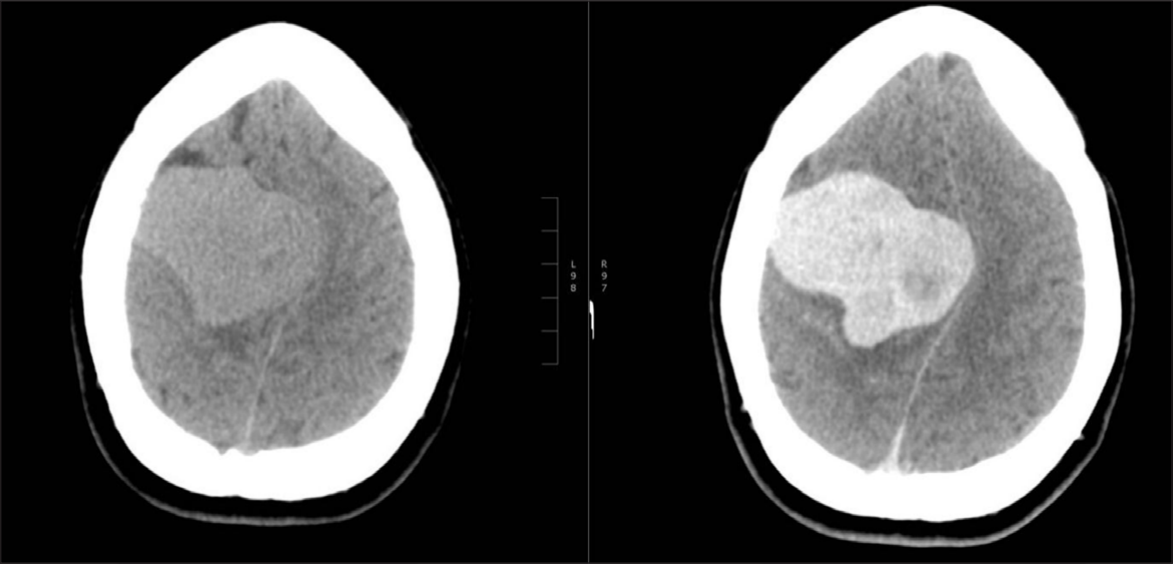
Plain CT and contrast-enhanced CT (CECT) show a lobulated, heterogeneously enhancing mass with several non-enhancing areas.
